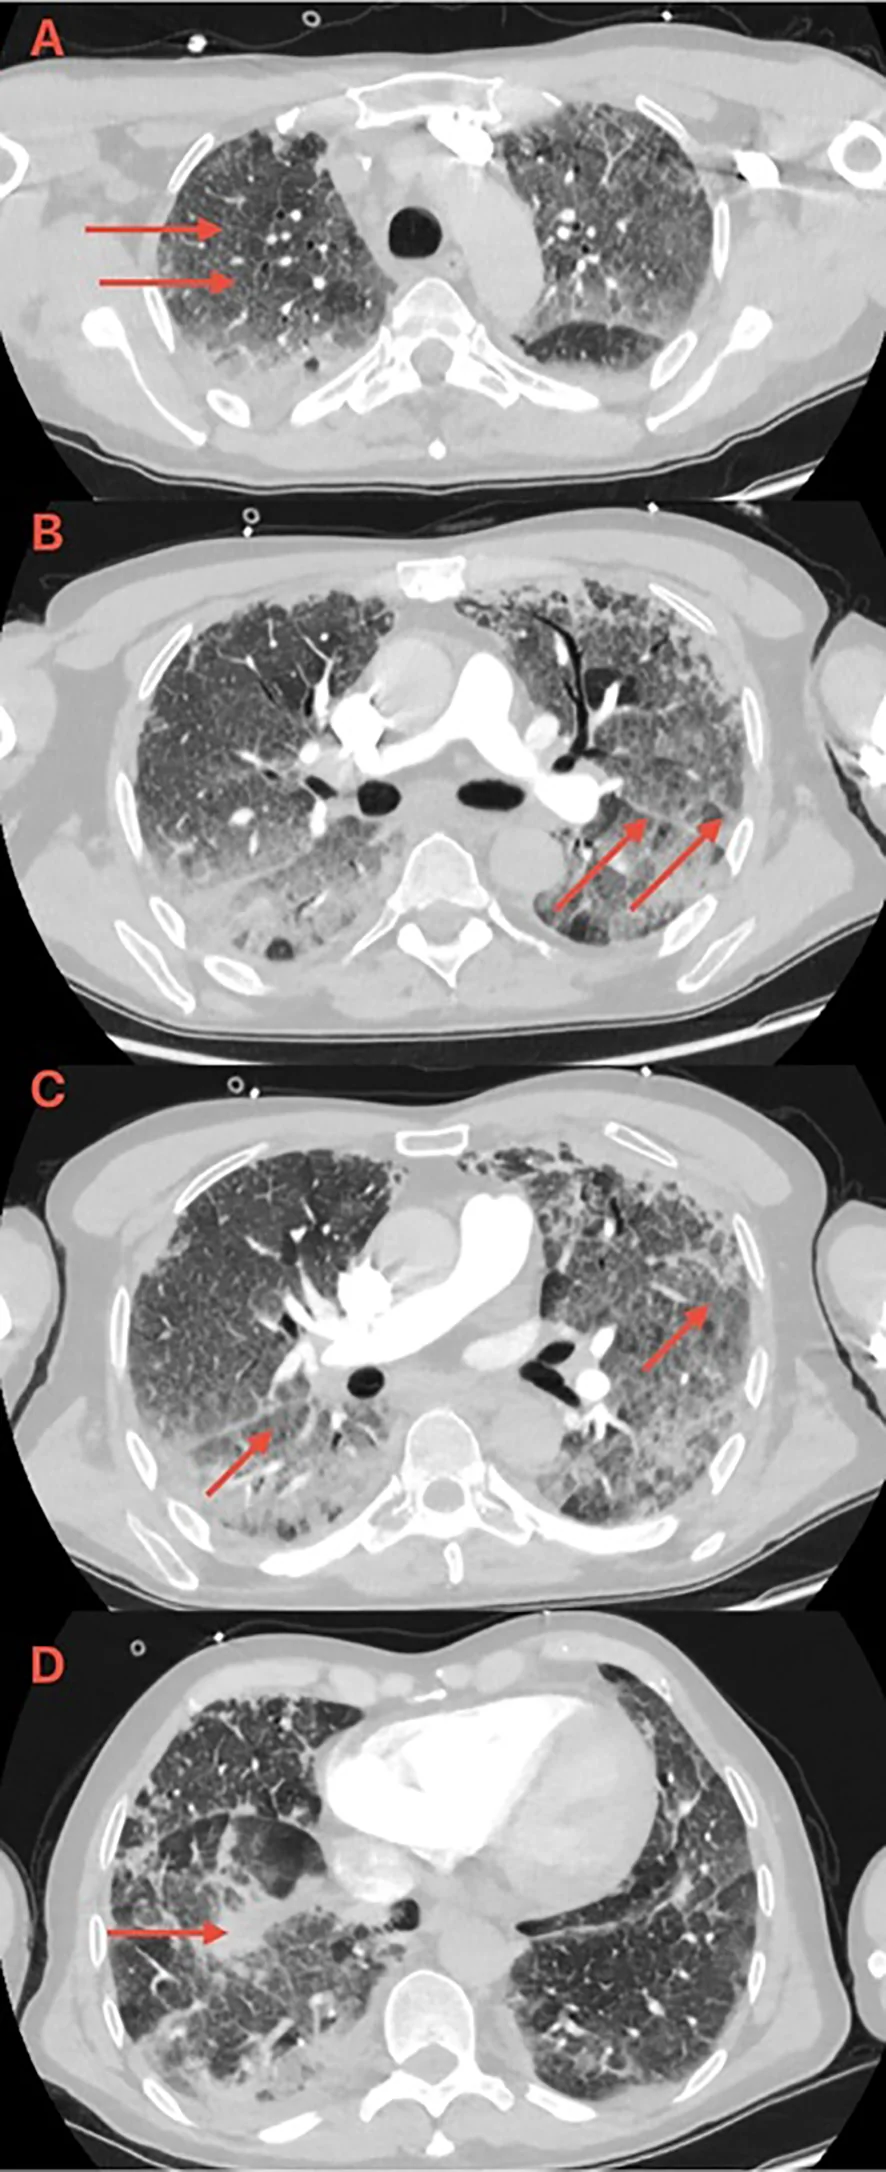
CT pulmonary angiogram on admission demonstrating features of the AIP pattern (red arrows): (A) nodular opacities. (B) interlobular septal thickening. (C) bilateral ground-glass opacities. (D) consolidations.
